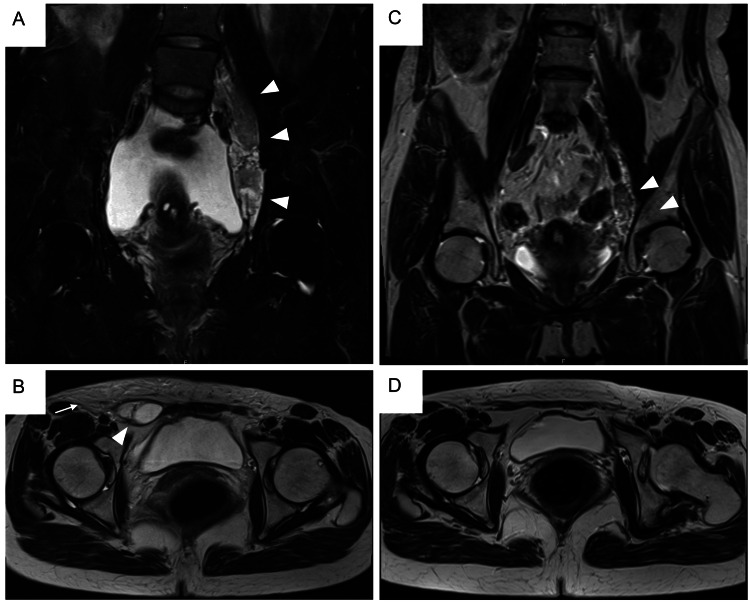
MRI images of the pelvic cavity The T2-weighted coronal (A and C) and axial (B and D) images are shown. A and B (February 2018): Pelvic retroperitoneal lymphangioleiomyomas (arrowheads in A) and lymphangioleiomyomas in the right groin area (arrowhead in B). Note lymphedema over the peri-groin subcutaneous tissue (arrow in B). C and D (August 2022): When the patient was on a dose of 0.5 mg/day of sirolimus, MRI images revealed remarkably shrunk pelvic retroperitoneal lymphangioleiomyomas (arrowheads in C) and almost complete resolution of lymphangioleiomyomas in the right groin area and lymphedema over the peri-groin subcutaneous tissue (D).
